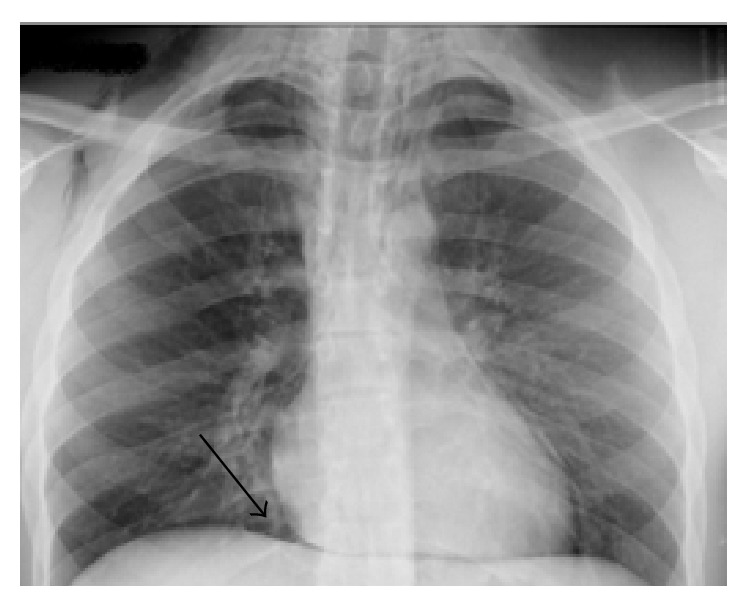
Posteroanterior chest radiograph showing subcutaneous emphysema and the continuous diaphragm sign (black arrow).
